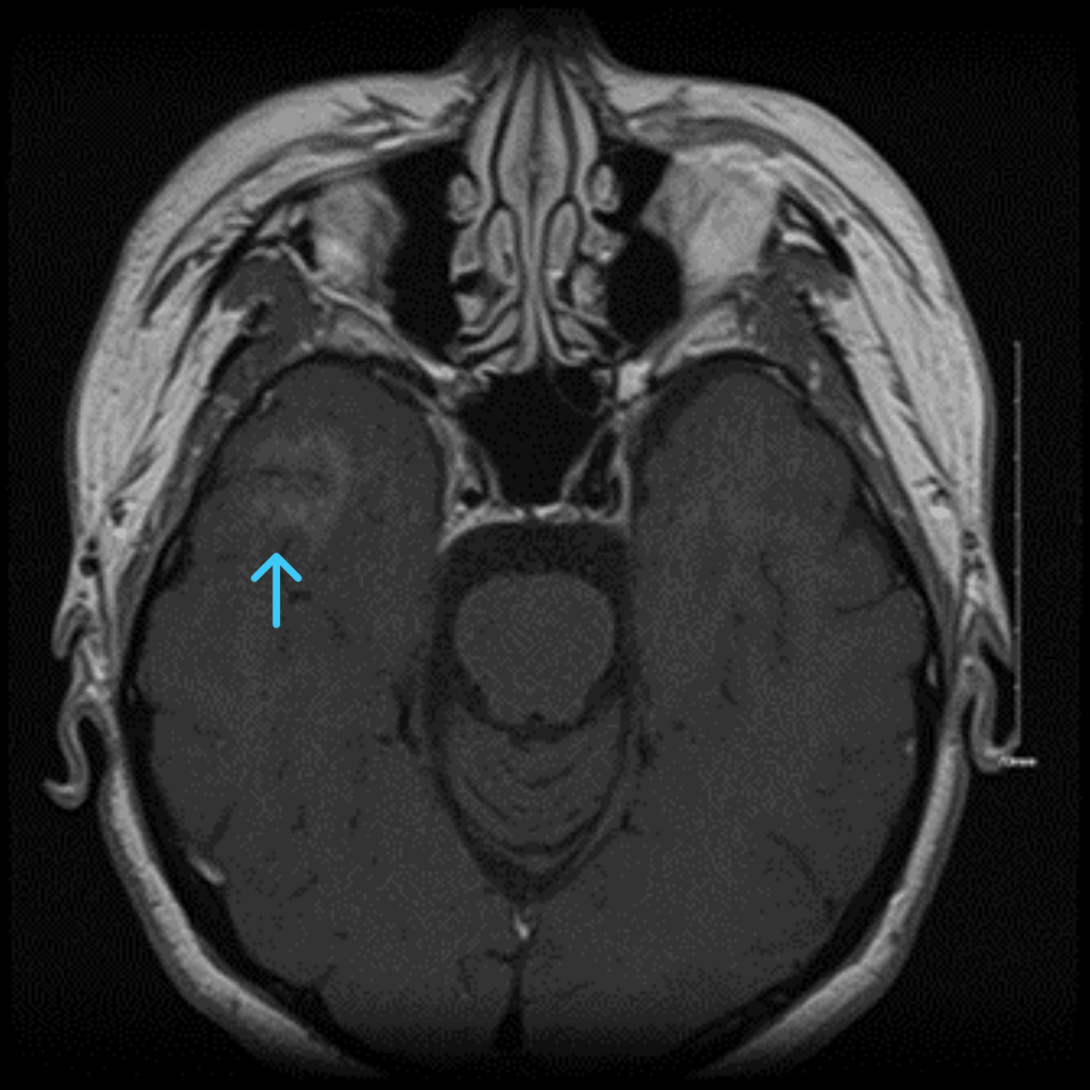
Focal right temporal lobe enhancement on post contrast axial magnetic resonance imaging
